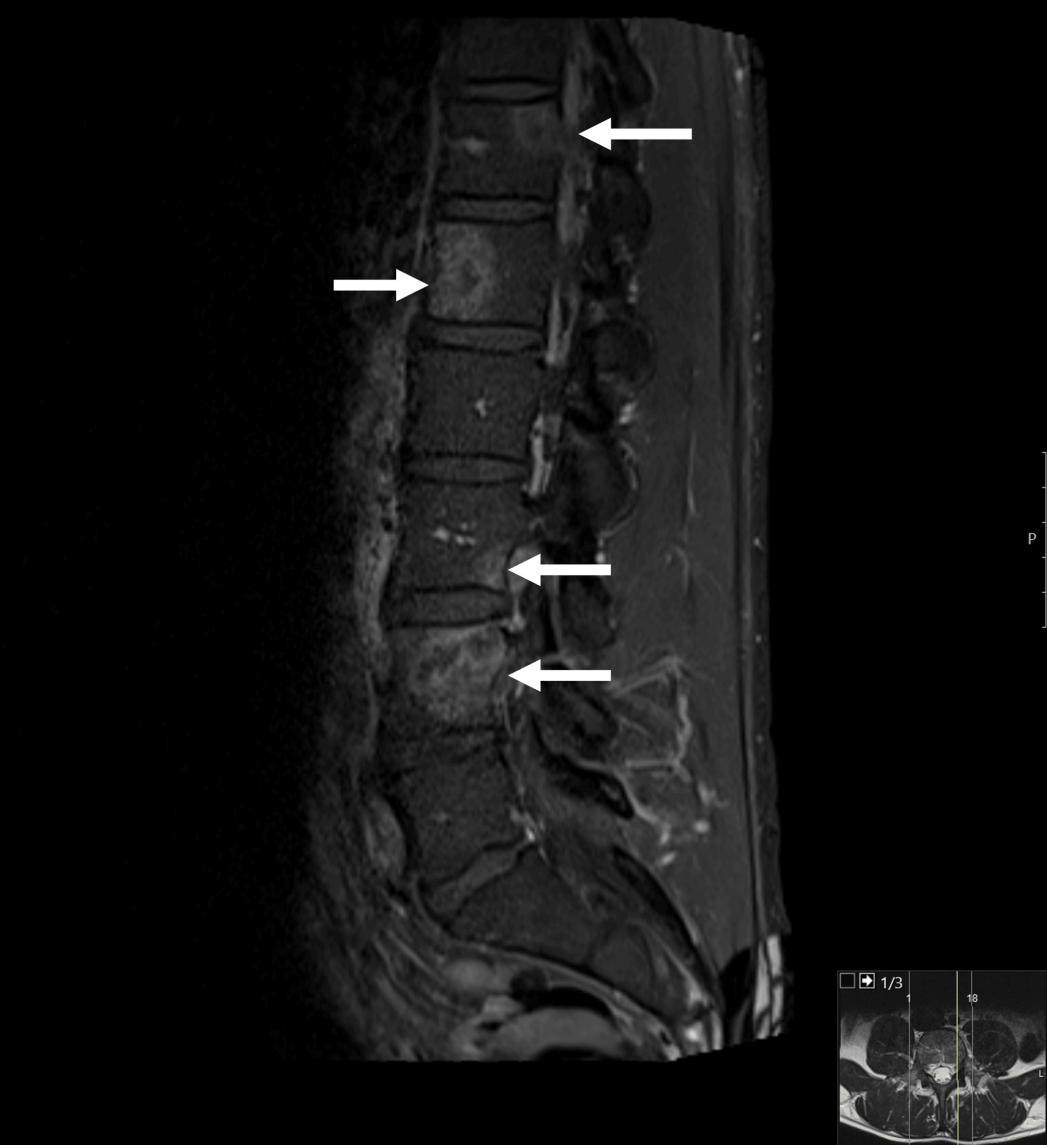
Magnetic resonance imaging T1-weighted imaging (MRI T1W1) sequence: sagittal plane Neoplastic invasion of the vertebrae of D12, L1, L3, and L4 (arrows)
